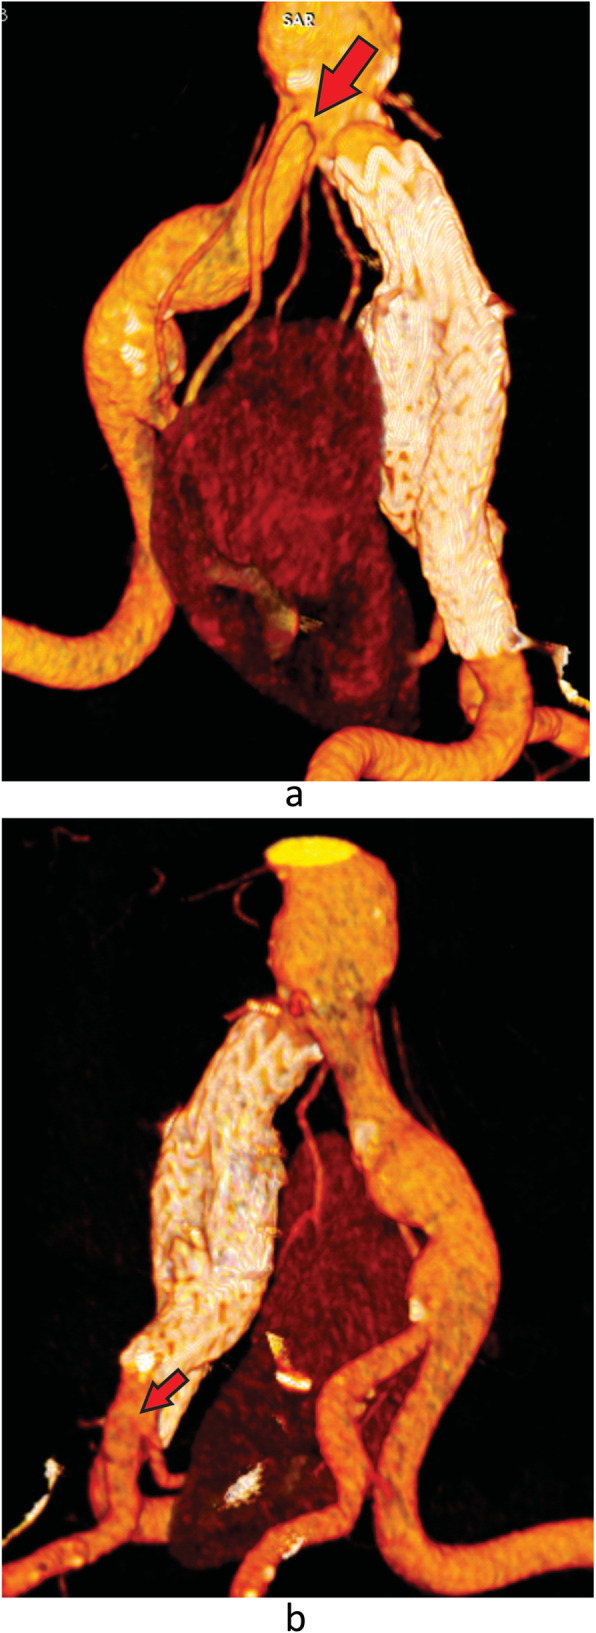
a 6 months postoperative three-dimensional reconstruction computed tomography showing proper positioning of the iliac branch device and preservation of the polar arteries. Red arrow. b 6 months postoperative three-dimensional reconstruction computed tomography showing proper positioning of the iliac branch device and preservation of the predominant renal artery. Red arrow
